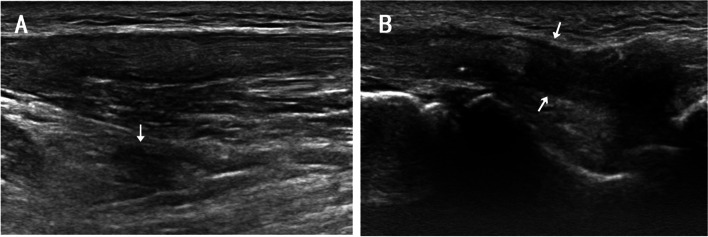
In ultrasonic images, extensor tendon of right ring and little finger was shown ruptured surrounding tissue edema, the proximal end (A) and the distal end (B) were indicated with white arrow
